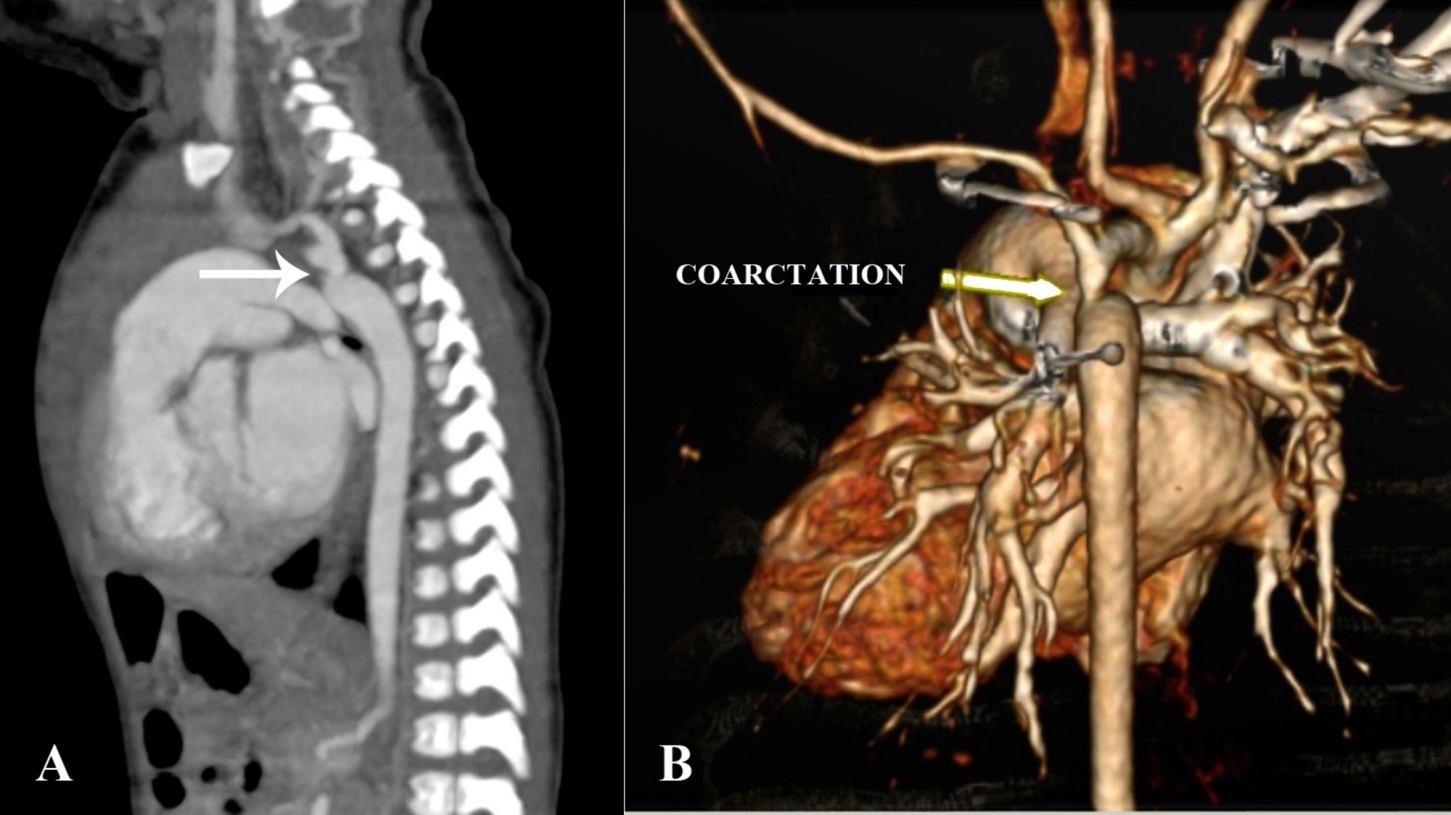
CT angiography sagittal (A) and 3D reconstruction (B) images Post-ductal coarctation of aorta (arrows) distal to the origin of the left subclavian artery.
